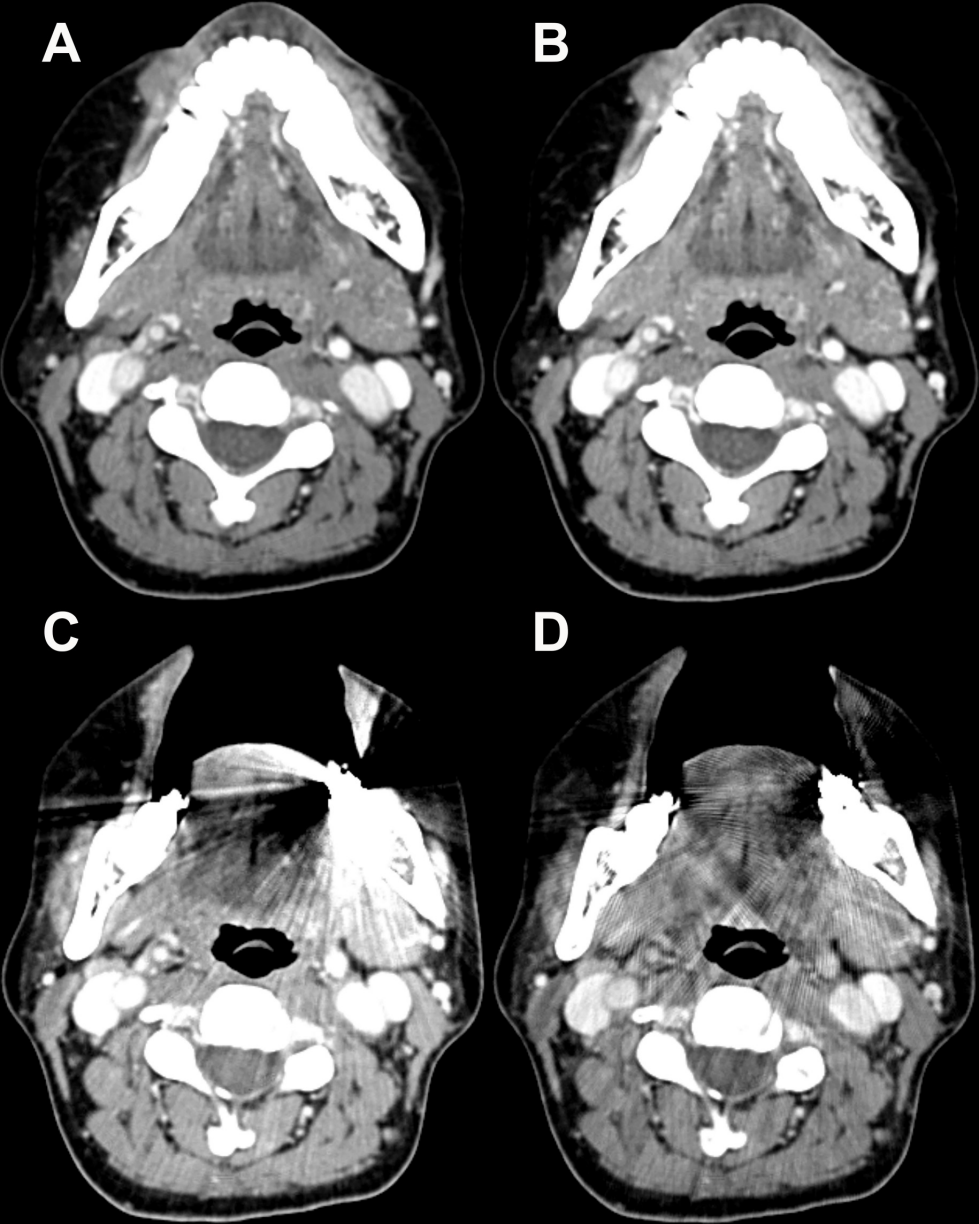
Post-contrast Computed Tomography (CT) images of a 51-year-old woman with a dental prosthesis. On a conventional CT image with the mouth closed (A) and on the conventional CT image with O-MAR processing (B), the mouth floor with extrinsic tongue muscles are well visualized without any artifacts. Contrarily, the structure is degraded by the metallic artifact from the lower molar prosthesis on the open mouth CT image (C). The streak artifact remains substantial even on the open mouth CT image with O-MAR processing (D). O-MAR = metal artifact reduction for orthopedic implants.
